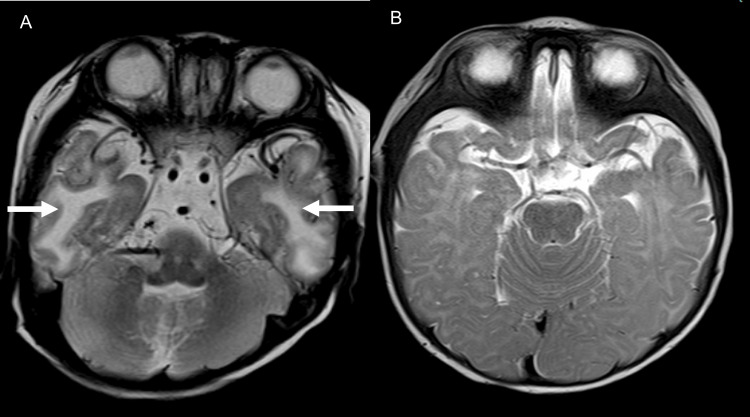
Case 1: T2-weighted image (A. T2-weighted image, B. T2-weighted image of a normal child of the same age). Compared to the normal case (B), high-signal intensity is shown in the subcortical white matter of the bilateral temporal lobes (A, arrows).
